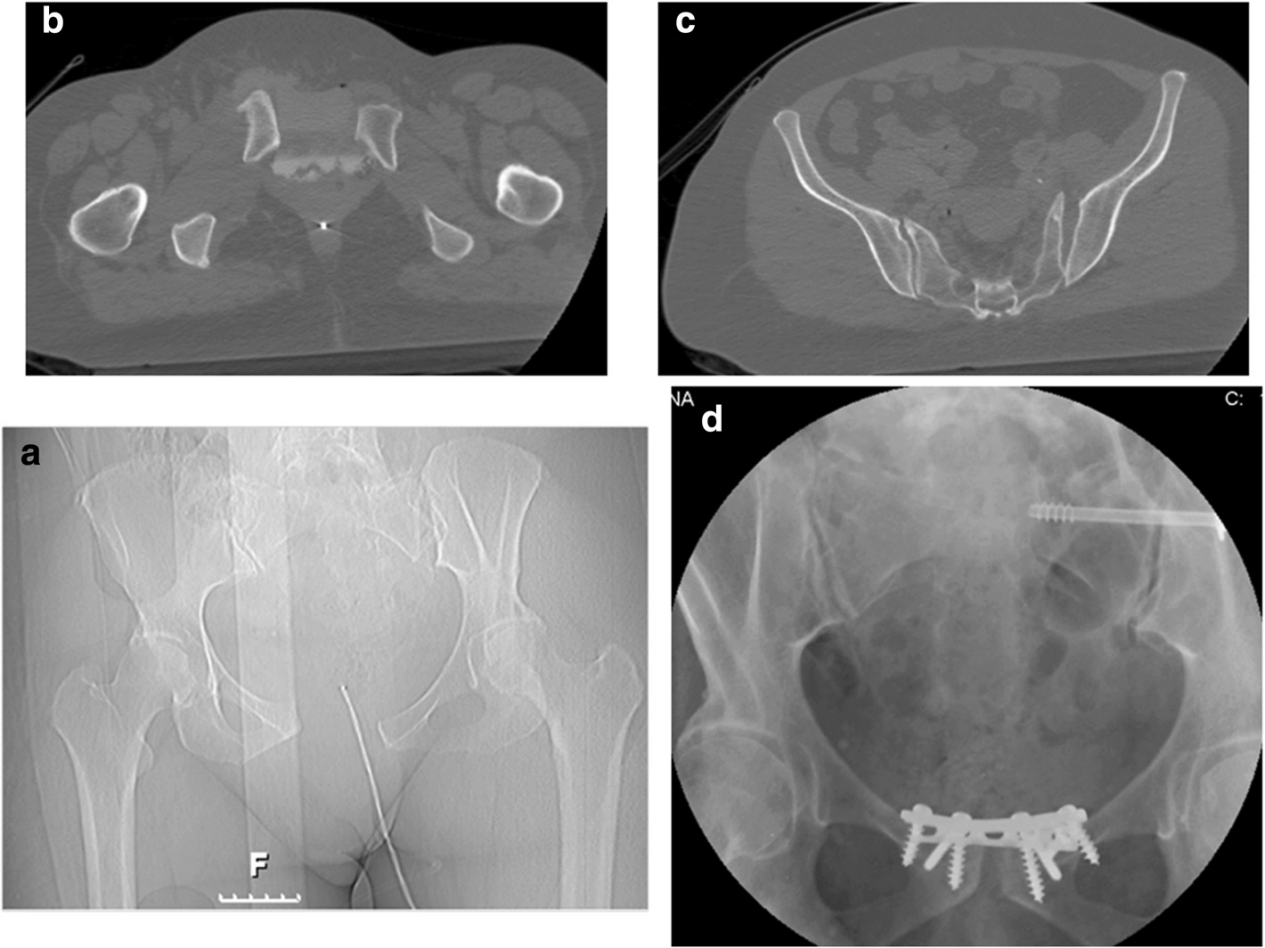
CT scan images showing the acute pelvic ring injury and AP pelvis post-operative x-ray. CT scan images of initial injury (November 2002). Scout image [a] shows the AP pelvic ring injury with diastasis of the symphysis pubis and left sacroiliac joint dislocation; [b] Axial image at the level of the symphysis pubis, showing diastasis of the symphysis pubis associated with extravasation of the contrast material from the bladder, indicating bladder injury; [c] Axial image at the level of the sacroiliac joint, showing widening and posterior dislocation of the left sacroiliac joint with rupture of the anterior sacroiliac ligaments; [d] AP pelvis image taken about six weeks post injury shows fixation of the symphysis pubis by two plates spanning the symphysis pubis and additional left iliosacral S1 screw. The symphysis is stabilized by seven screws; four 6.5 mm diameter cancellous screws and three 4.5 mm diameter cortical screws. The iliosacral screw is a 7.3 mm diameter cannulated screw with a washer flush to the outer table of the ilium, indicating compression between the screw and the bone
